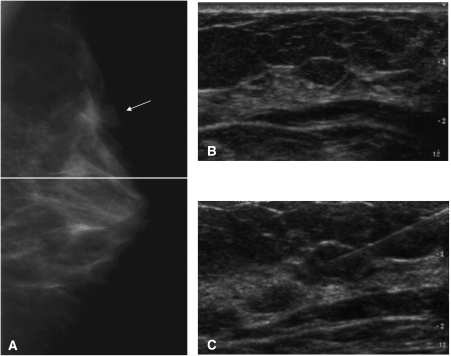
Typical imaging features of a probably benign, circumscribed, discrete mass, graded 3B in our programme. (A) Mammogram, left lateral view, with the arrow pointing to a circumscribed mass. (B) Breast ultrasound of the lesion, showing a circumscribed oblong mass. (C) Ultrasound image, documenting the presence of the biopsy needle within the lesion.
